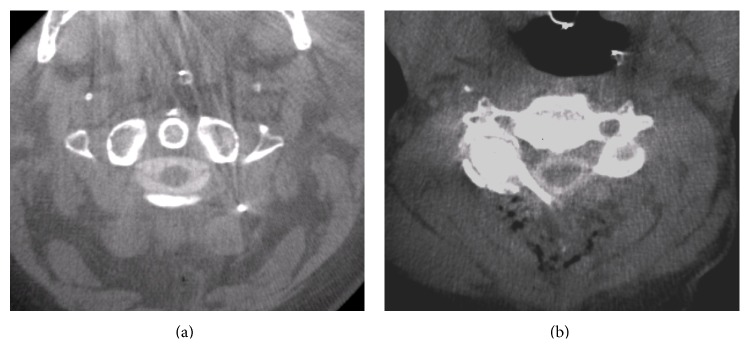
Without metal artifacts, good quality images can be obtained comparable to CT myelography. Transverse views of case 4 (a) and case 8 (b).
